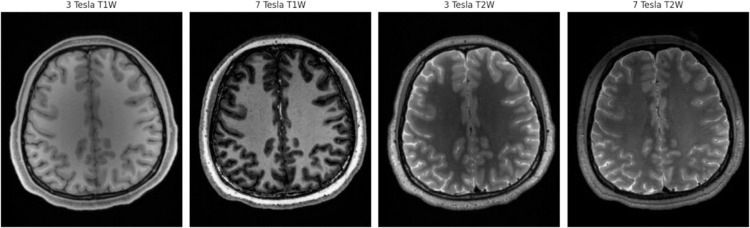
Different MRI scan contrasts and strengths provided by the paired dataset [15].
